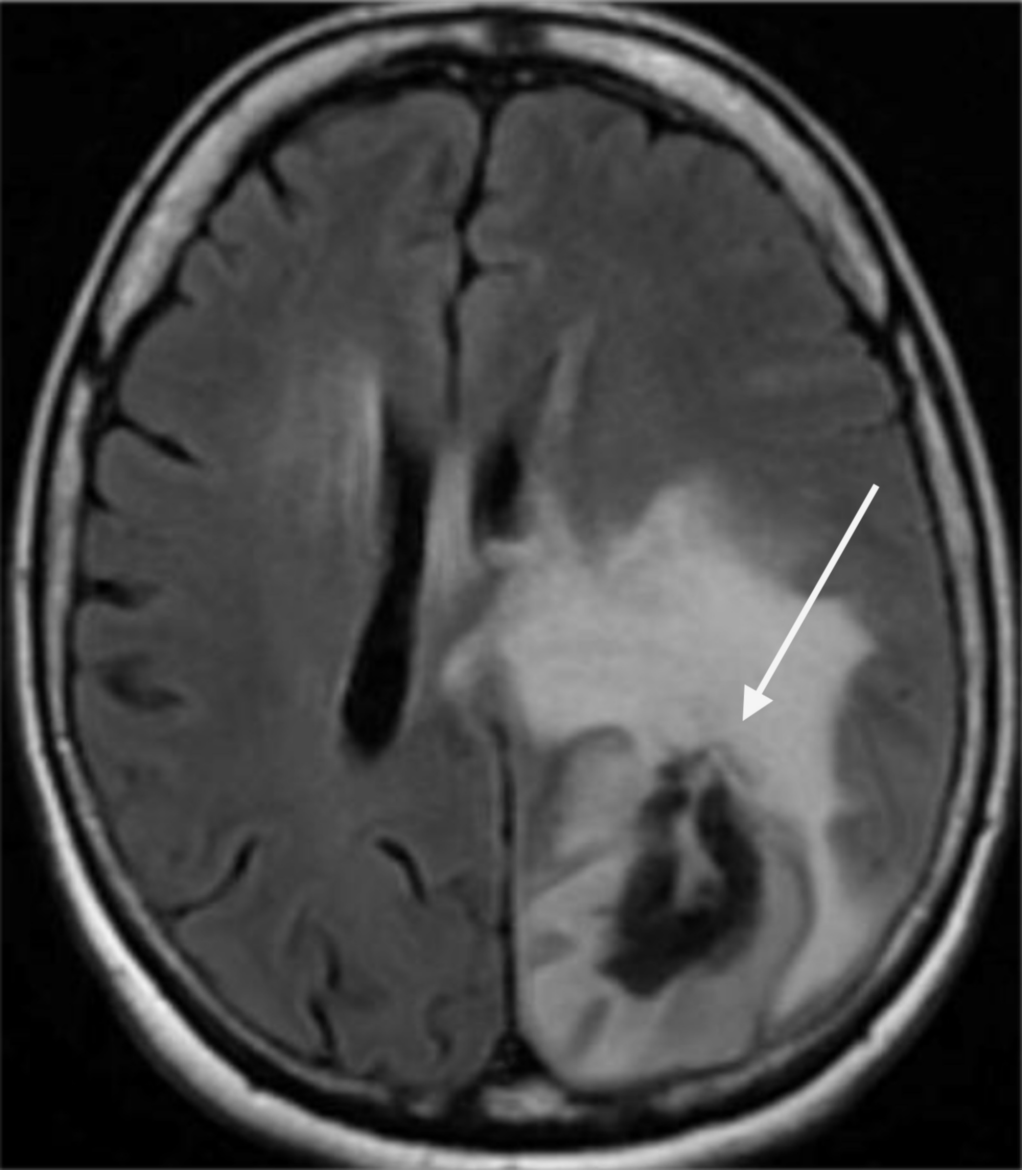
MRI of the brain. MRI of the brain showing a hemorrhagic mass within the left parieto-occipital lobe measuring 5.2 cm x 2.6 cm x 3.6 cm in size with surrounding vasogenic edema.
